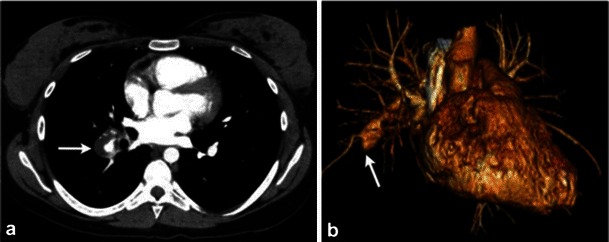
Contrast-enhanced axial CT (a) in a patient with Bechet’s disease demonstrate a focal aneurysm of the right lower lobe pulmonary artery with eccentric mural thrombus (*arrow*). Volume rendered image (b) better depicts the eccentric saccular aneurysm (*arrow*). This patient underwent right lower lobectomy for recurrent haemoptysis
